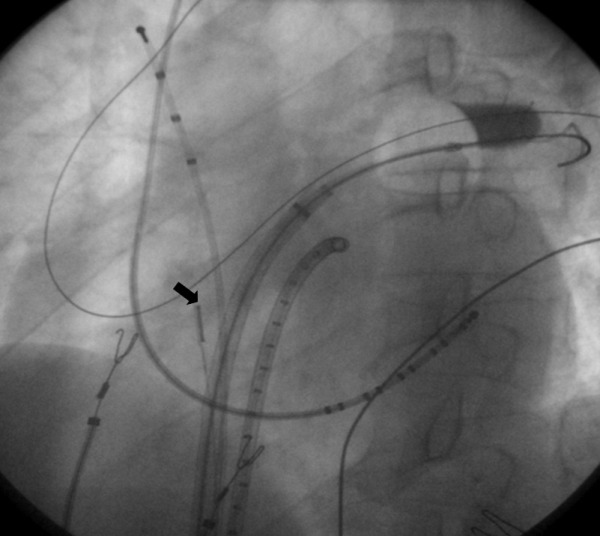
Angiographic occlusion of left upper pulmonary vein: contrast retention within the pulmonary vein ostium. Two transeptal sheaths are inserted within the left atrium. The black arrow shows the intracardiac echocardiographic catheter located in the right atrium with the optimal tilt and orientation necessary to visualize the left pulmonary veins.
